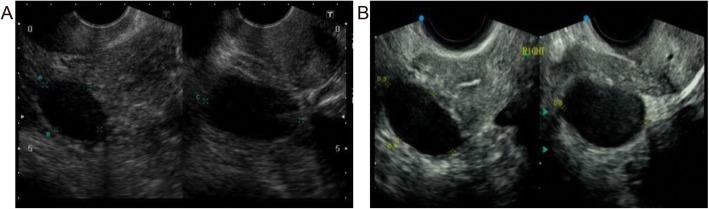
The sonographic findings of the uterine EOBTs. A hypoechoic mass measured 5.2 x 5 x 4.7 cm was detected incidentally 2 years ago (a). The lesion was indolent, approximately 5.8 cm in greatest diameter (b)
